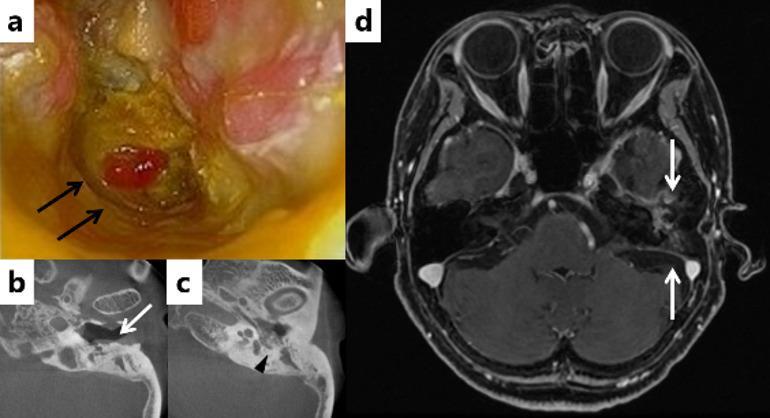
Malignant otitis externa. a Otoscopy, b–c CT, d contrast-enhanced MRI. A woman in her 70s with type 2 DM presented with left ear leakage and pain. Otoscopy (a) reveals the exposed bone in the wall of the external auditory canal (arrow). Axial CT (b and c) shows soft-tissue thickening of the external auditory canal and tympanic cavity (arrow) with focal erosion of the temporal bone (arrowhead). Contrast-enhanced MRI (d) demonstrating marrow edema and enhancement extending toward the skull base. These findings reflect invasive infections in immunocompromised patients with diabetes and warrant urgent treatment. Abbreviation: CT, computed tomography; MRI, magnetic resonance imaging; DM, diabetes mellitus
